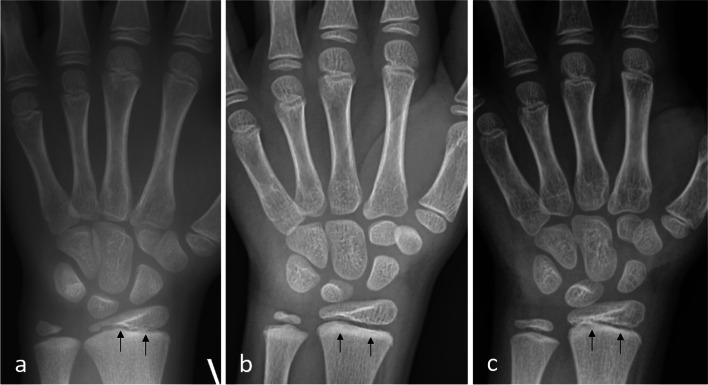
a-c Radiographs of the wrist in 3 females aged 6-7 years. Conspicuity/visibility of the zone of provisional calcification in radius only (ZPC) was scored as (a) not visible, (b) moderately  visible or (c) mildly  visible
